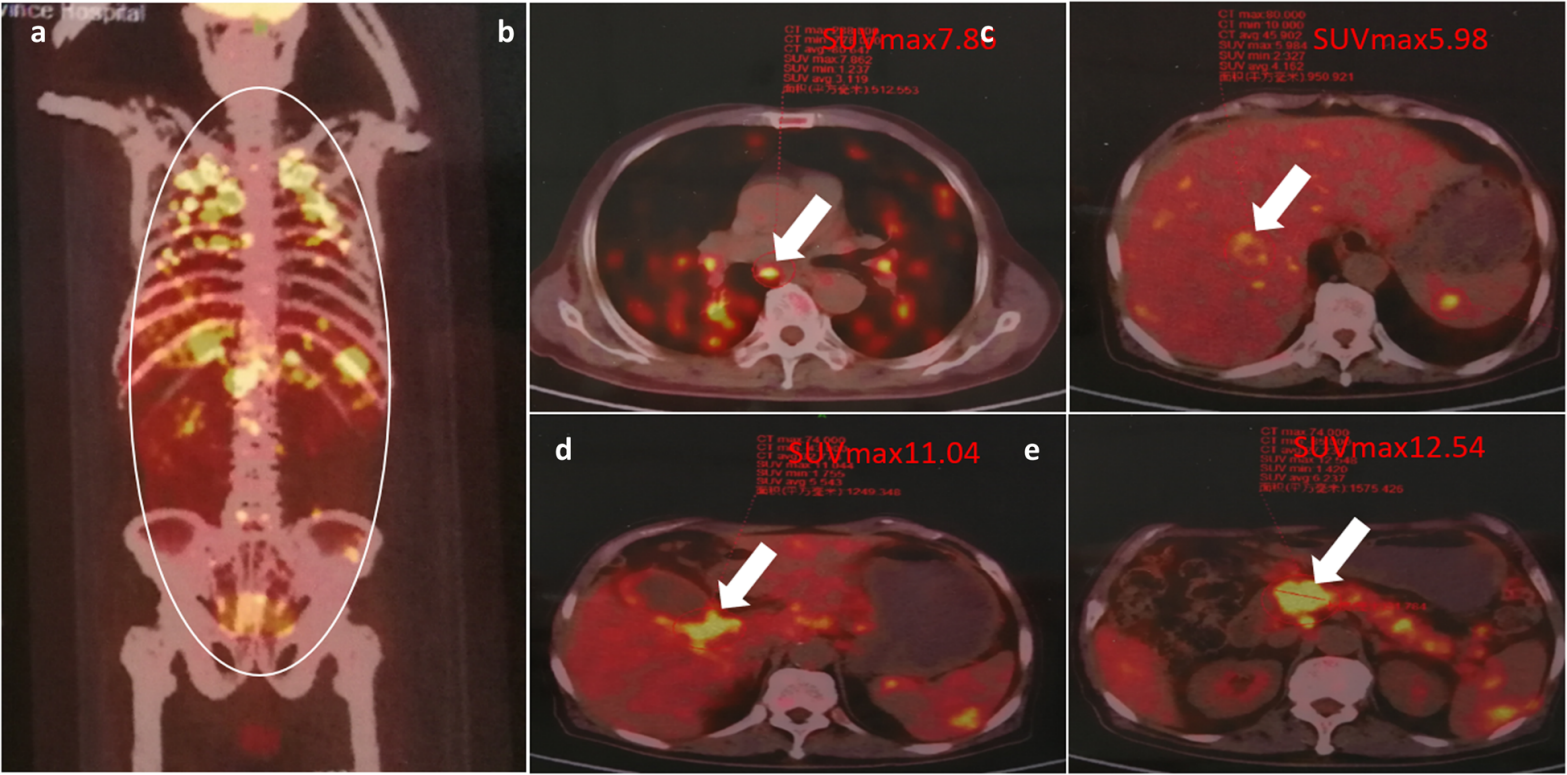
FDG-PET showed high uptake at different foci. a Foci in the whole body were defined by a circle. The yellow bright spots represented high uptake of ^18^F-FDG by local tissues. The maximum standard uptake value in different organs were shown, including lung (b, 7.86), liver (c, 5.98), gallbladder neck (d, 11.04), pancreatic head mass (e, 12.54)
